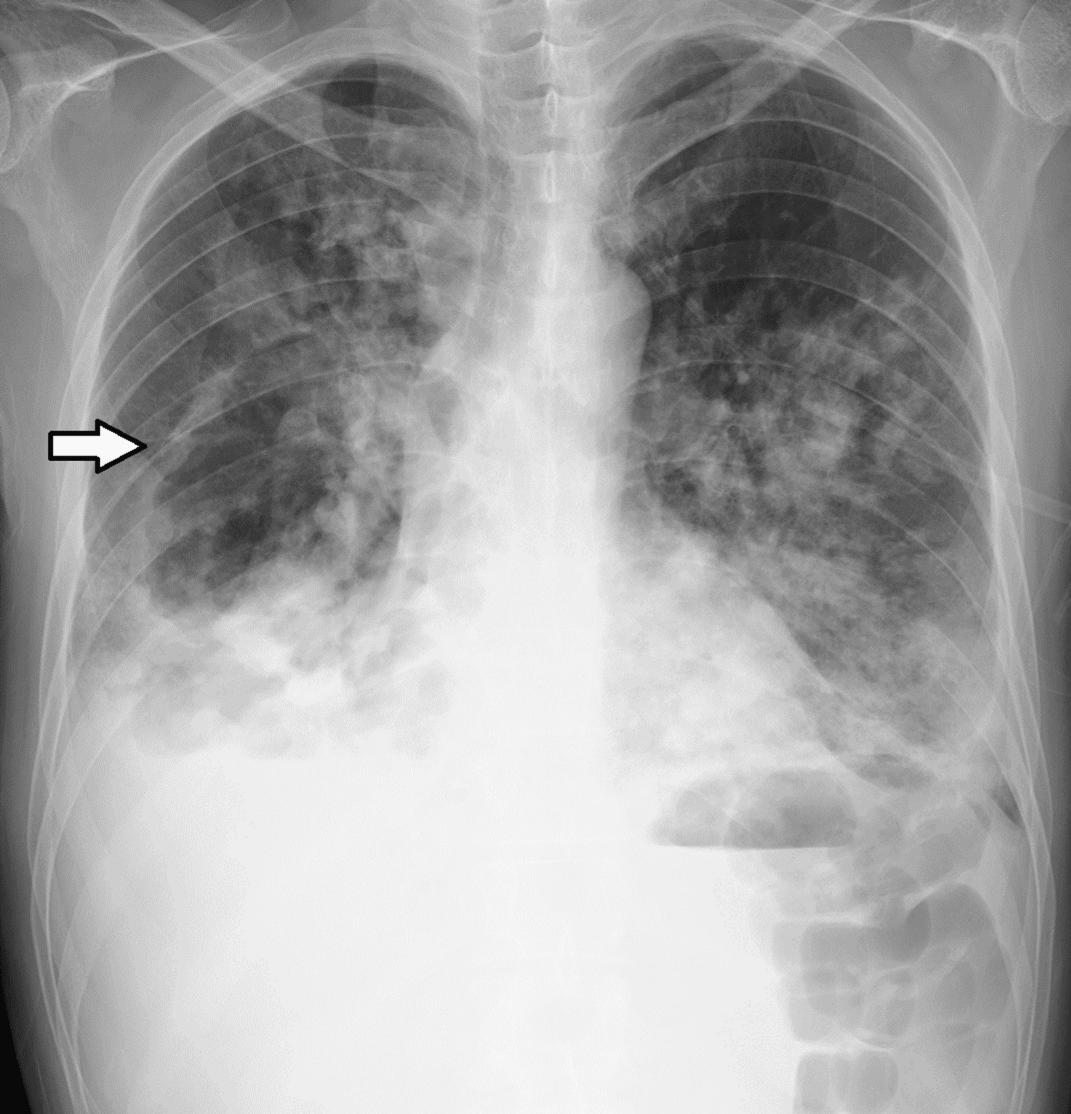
Chest radiograph on admission The radiograph shows a cavitary shadow in the right middle lung field (arrowhead) and diffuse infiltrative shadows in the right upper lung field as well as the left middle and lower lung fields.
